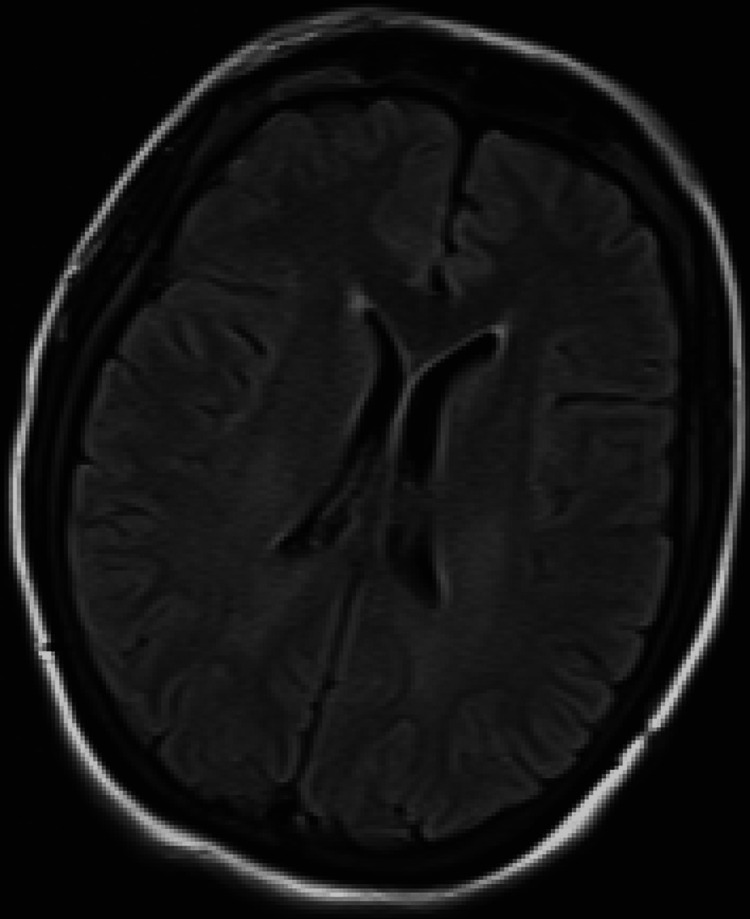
MRI head without contrast demonstrating no evidence of infarction, mass, or lesion as a potential cause of the patient’s symptoms.
